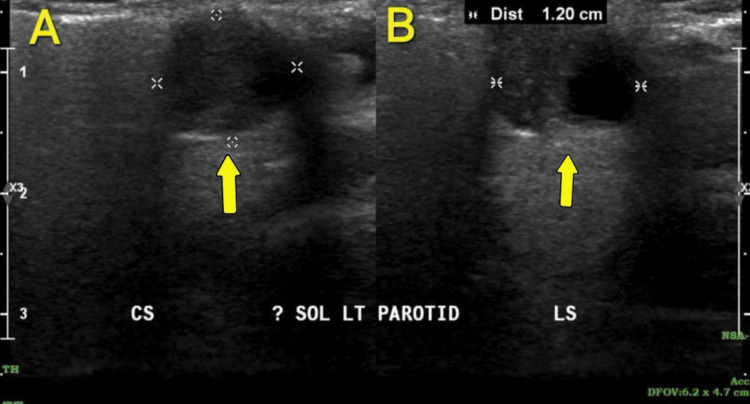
Ultrasound of the left parotid gland A: the coronal section (CS) shows a well-defined solitary hypoechoic lesion in the parotid gland; B: the longitudinal or transverse section (LS) also shows a hypoechoic lesion in the superficial lobe of the parotid gland.
